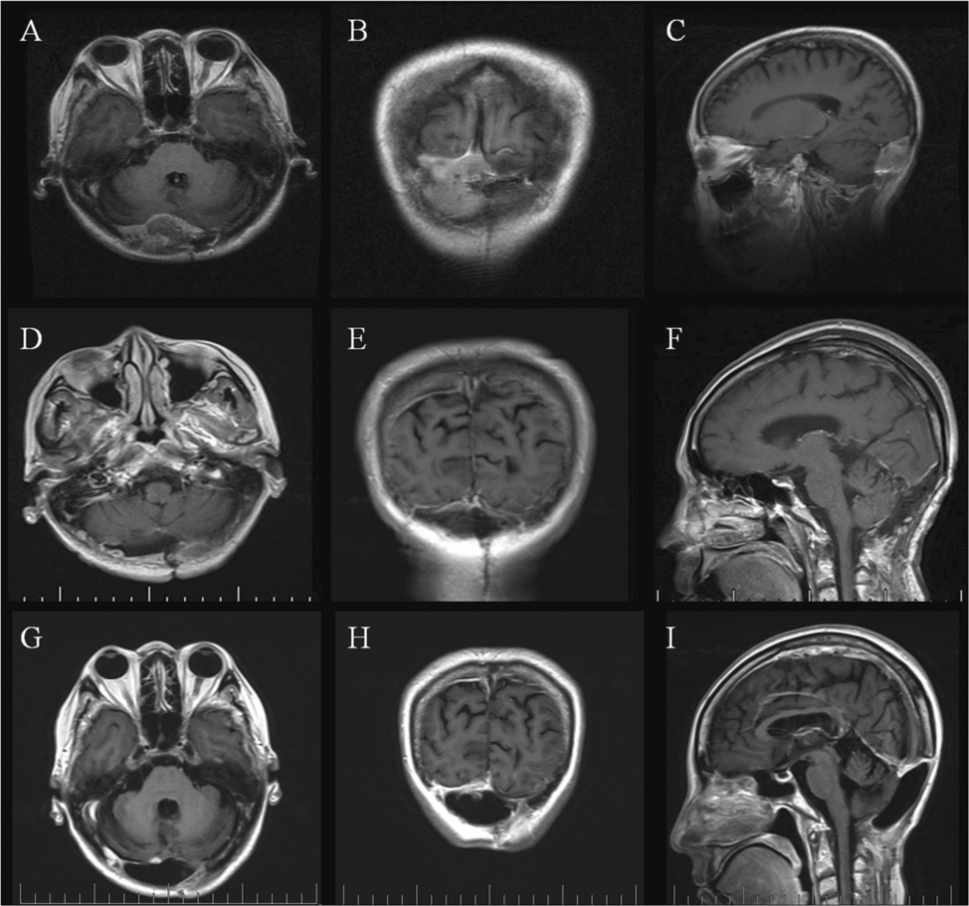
MR images showing the MFH of the posterior cranial fossa in preoperative and postoperative follow-up. A, B, C) MR images showing an expansive mass of the posterior cranial fossa with an irregular outline involving the right cerebellar hemispheres, posterior fossa dura, occipital bone and the torcular herophili. The signal intensity of the lesion is slightly hyperintense on the T1-weighted Gd-enhanced image. T1-weighted Gd-enhanced image showing moderate heterogeneous enhancement throughout the lesion. D, E, F) Postoperative early stage MR images showing no residual mass lesion. G, H, I) Follow-up MRI on the postoperative sixth month showing tumor non-recurrence. MR, magnetic resonance.
